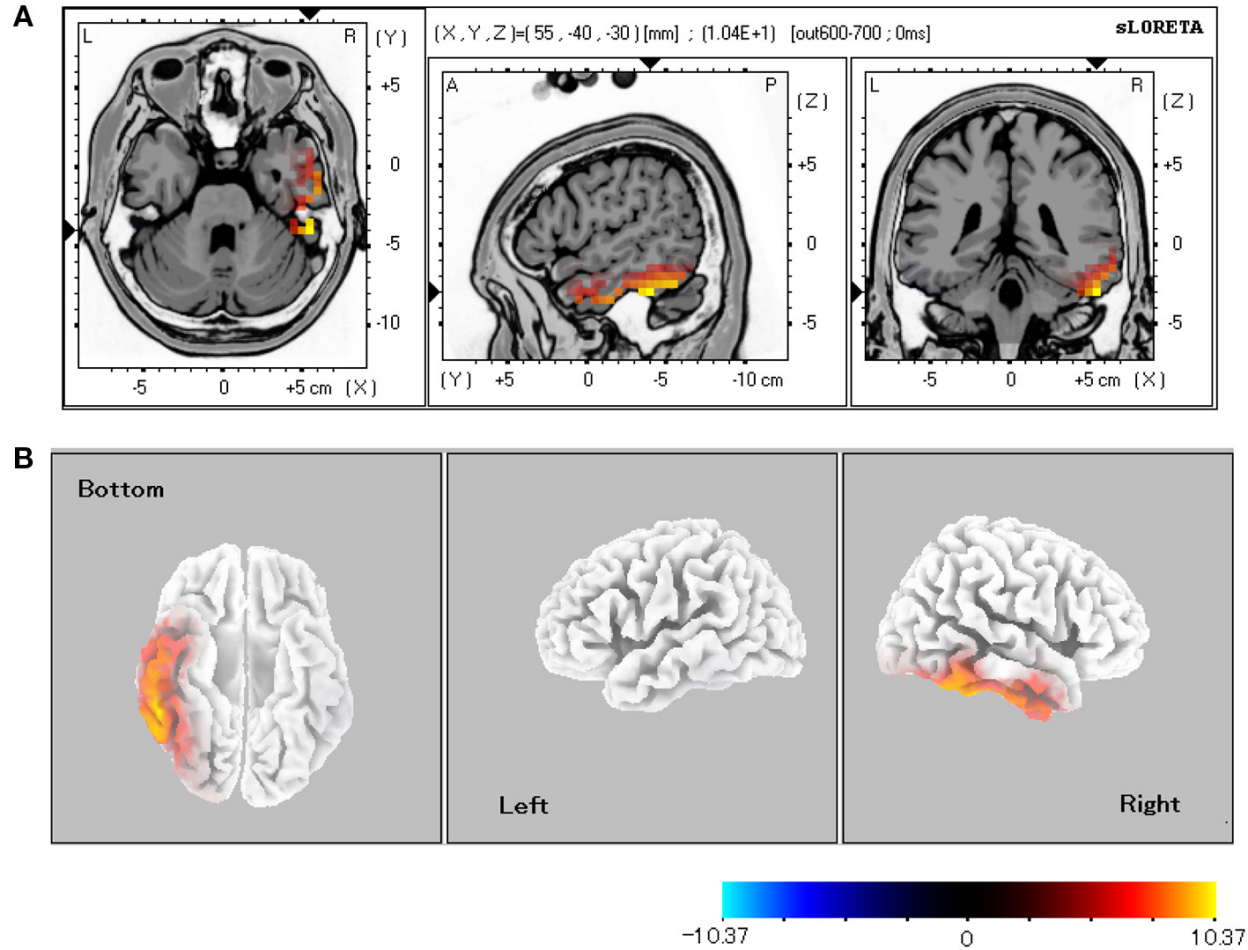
sLORETA comparison of source localizations between the CS+ and CS− conditions. (A) MRI planes (Colin27 brain) in the axial, sagittal, and coronal views and (B) cortical maps from the bottom, left, and right sides of the average among the 600–700-ms latencies (CS+ vs. CS−). The statistically significant regions acquired from all the subjects were displayed on a standard brain template in (A). The source estimation indicates the significant roles (*p* < 0.05 for all the subjects) of the right lateral fusiform gyrus.
